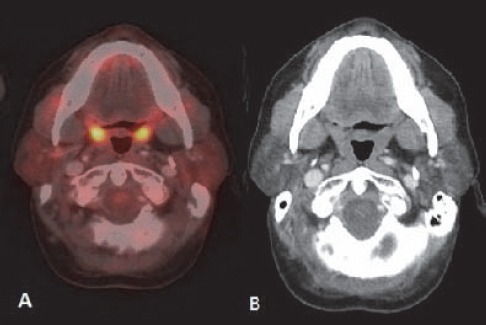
Physiological distribution of radiotracer in bilateral tonsil. No obvious abnormality in corresponding CT scan images (B). Compare with Figure 1. Corresponding change is seen in CT scan in case of pathological radio tracer uptake
